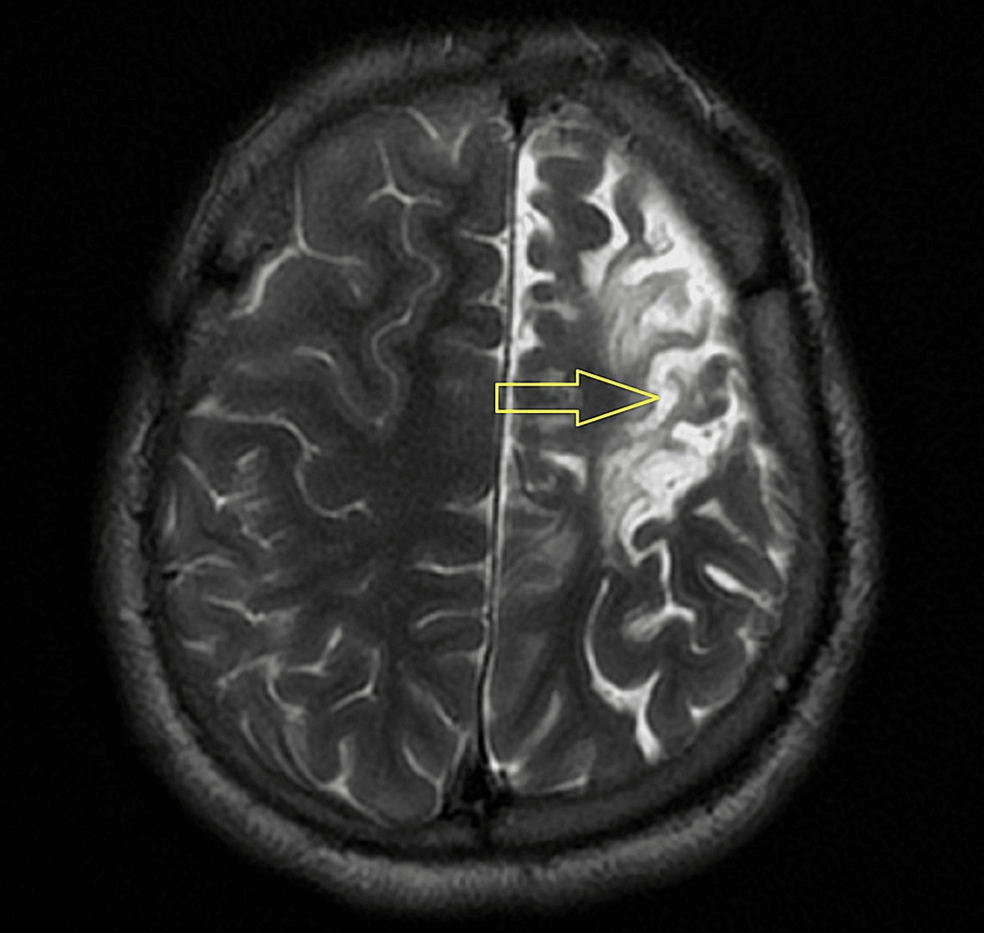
Axial T2 weighted MRI of the brain showing atrophic changes with volume loss involving the left cerebral hemisphere (yellow arrow). MRI: magnetic resonance imaging
